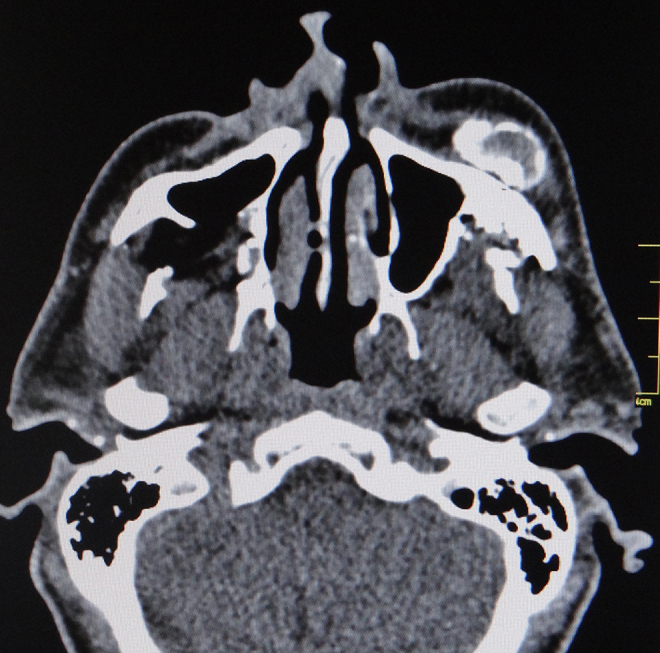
CT scan. Identification of the largest lesion, with a radiolucent interior and compact bone periphery and bulging of adjacent soft tissues.
